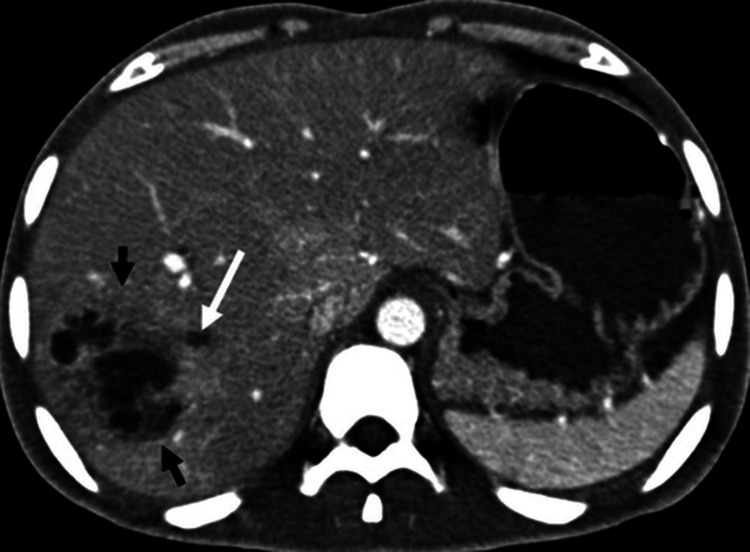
CT image showing multiple round to oval peripherally enhancing hypodense lesions in the liver, suggestive of pyogenic liver abscess
